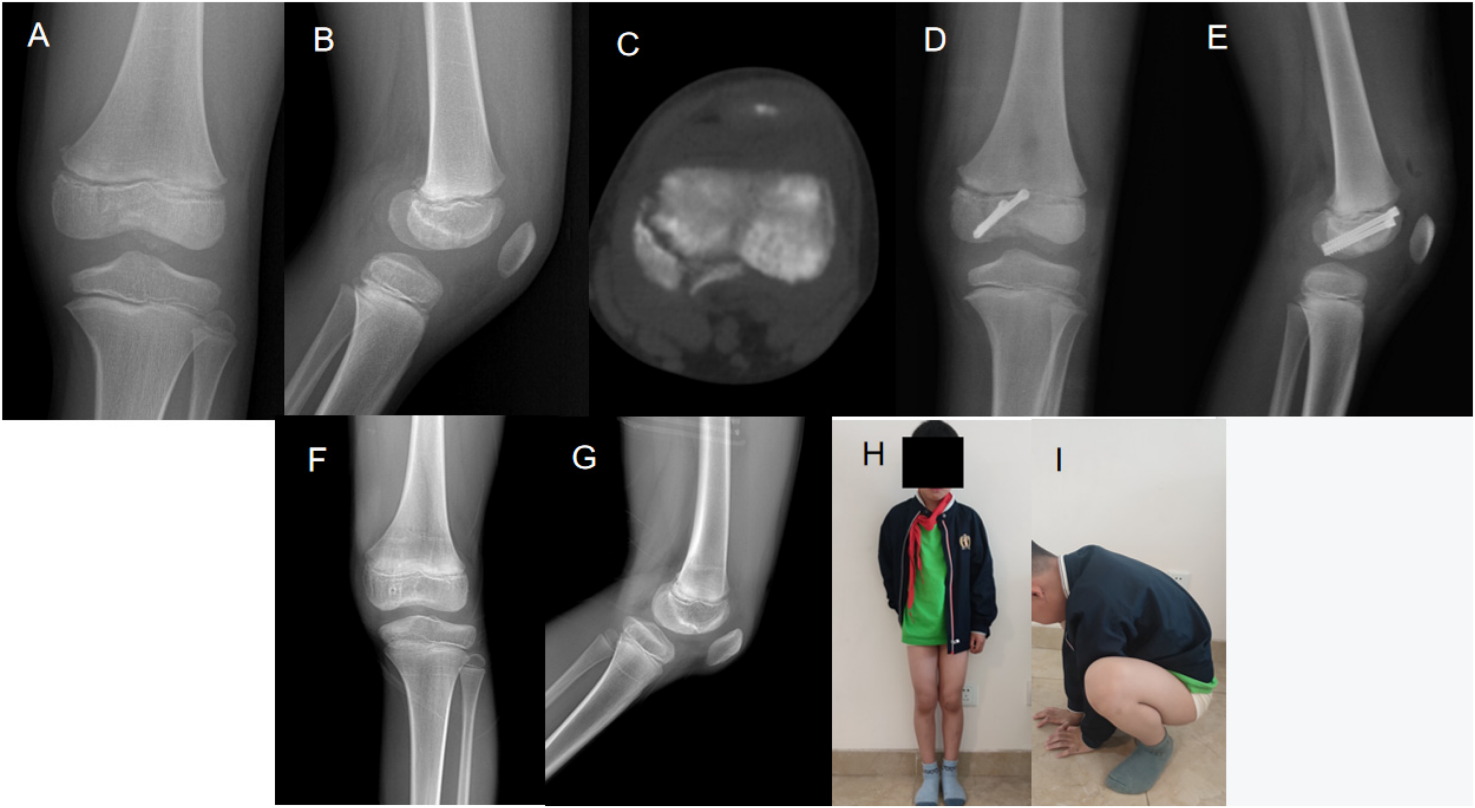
A 7.2-year-old boy with left medial femoral condyle fracture from a vehicle accident: (A) X-rays preoperative showing fracture; (B) CT exam preoperative for the evaluation of injury; (C) Postoperative images showing two 4.0 mm screws for fixation; (D) Two years post-operative X-rays showing healing process; (E) Postural images 18 months post-surgery showing functional recovery; (F, G) Post-operative X-rays showing alignment; (H, I) Clinical images showing functional results and mobility.
